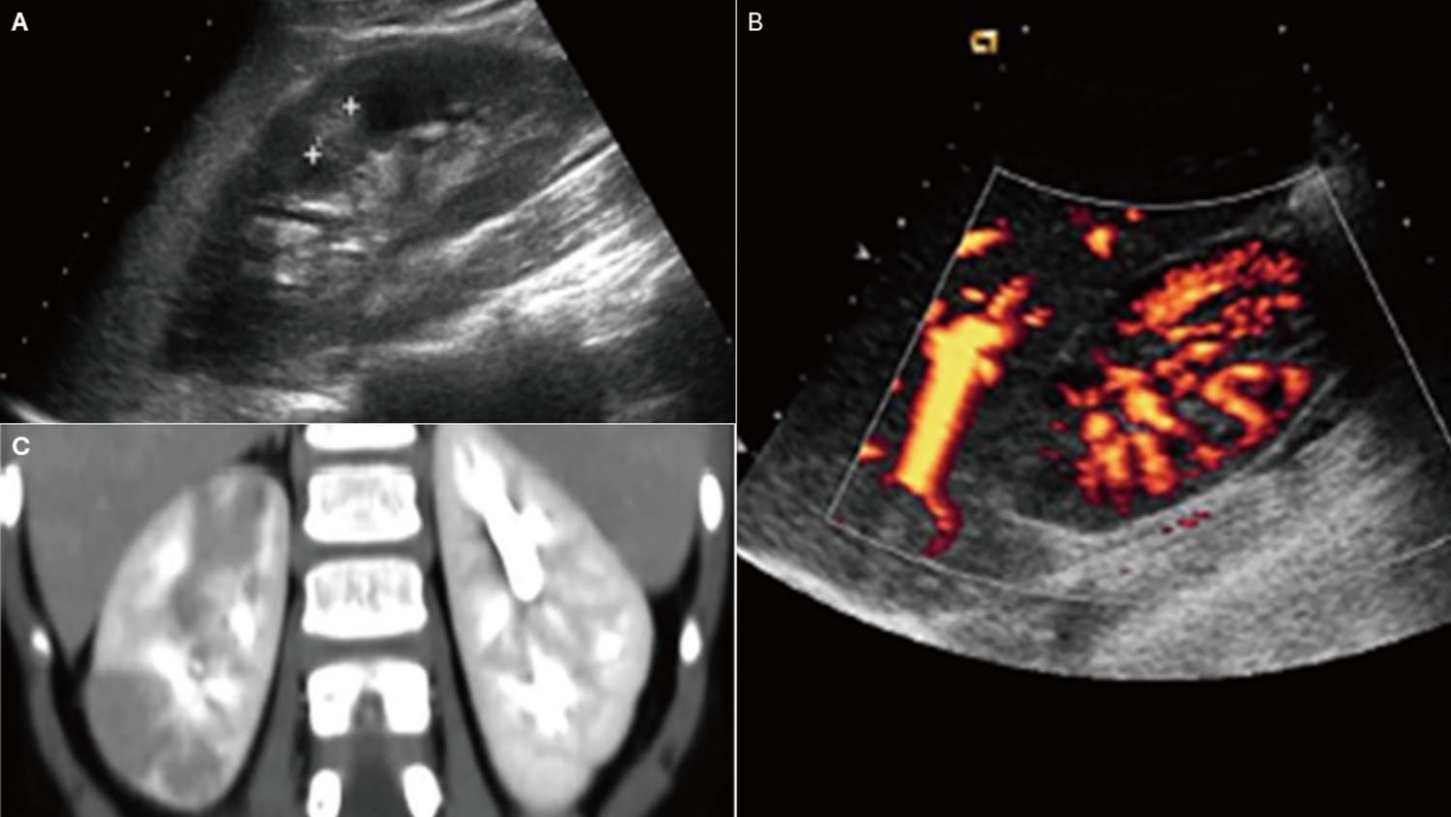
Radiological images of AFBN. A Abdominal US reveals a hyperechoic mass in the mid-zone of the kidney. B Abdominal US with power-Doppler analysis shows hypoperfused areas in the upper pole of right kidney. C Contrast-enhanced CT scan of the abdomen reveals multiple hypodense, non-enhancing lesions in the right kidney. Adapted from refence [30], reproduced under Creative Commons License CC BY. Ultrasound, US; computed tomography, CT
